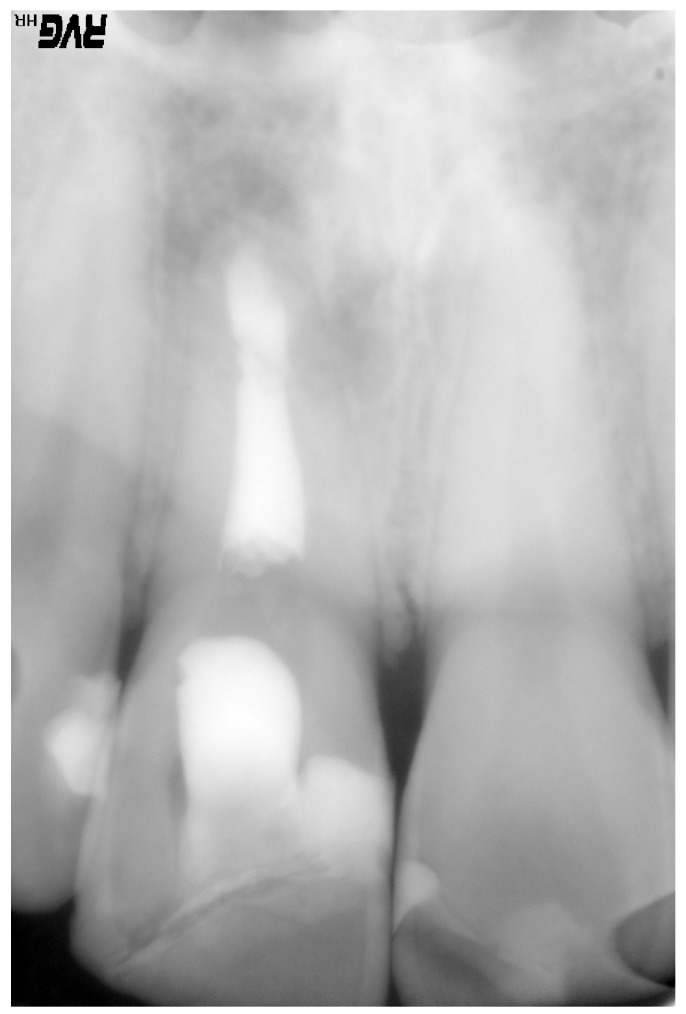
Postoperative control following periapical surgery.
